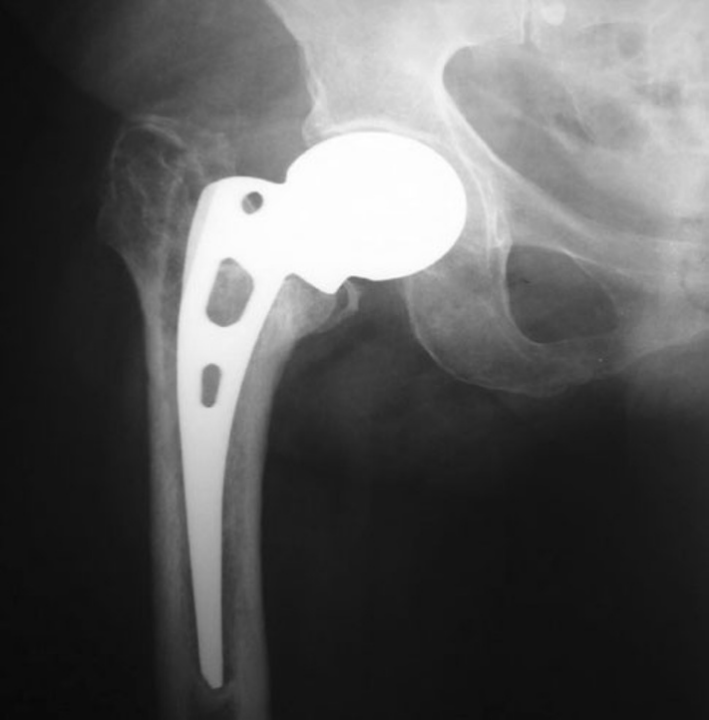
A 64-year-old patient with fracture neck femur treated with Austin Moore arthroplasty. Two years post-operatively radiograph showed subsidence of the prosthesis
